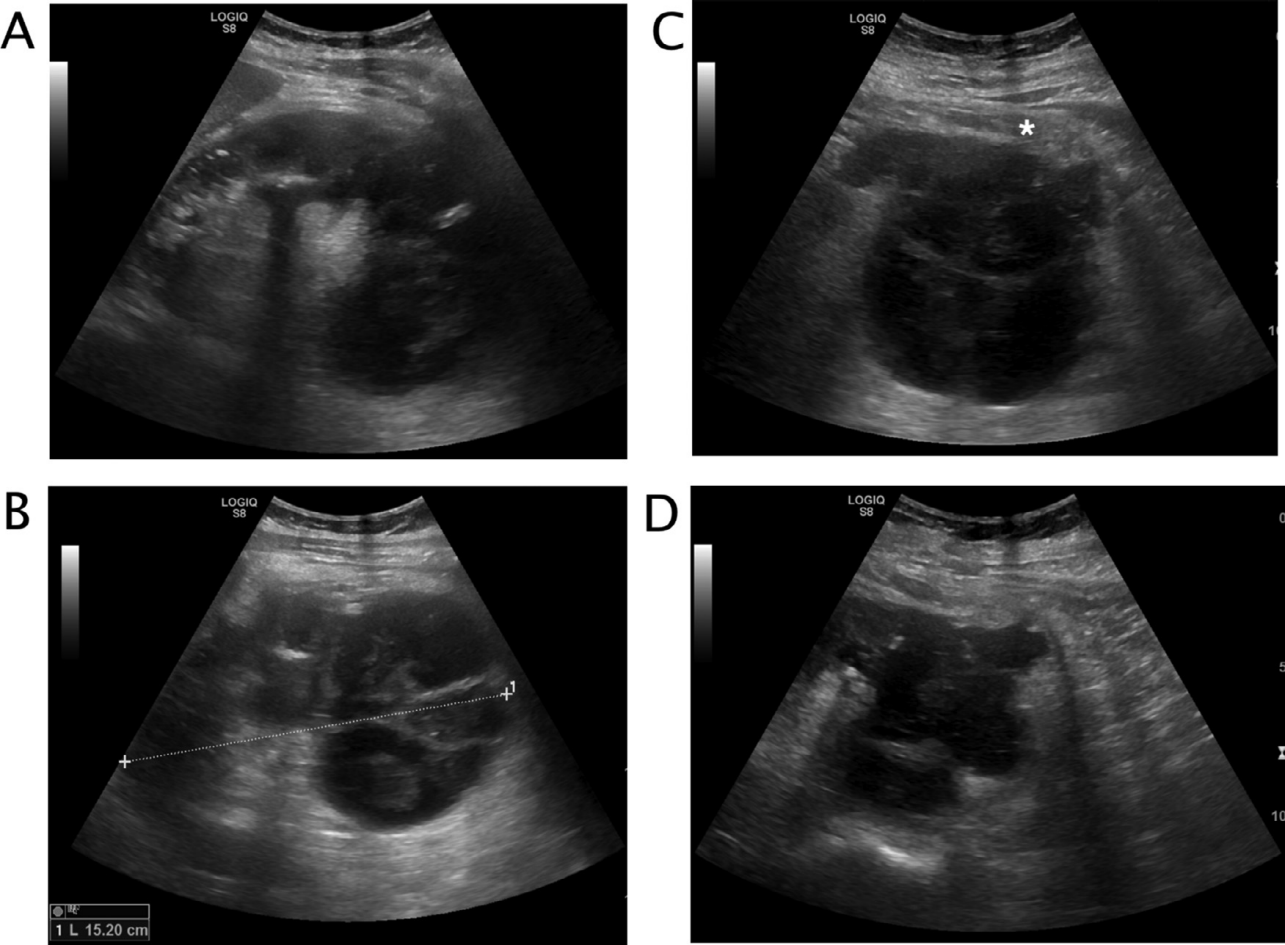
Left kidney ultrasound. The kidney is enlarged (A, B) with fluid filled dilated calico-pelvic system, within inhomogeneous hypoechogenic material is appreciable (A, C). Hyperechogenic spot are visible, referable to calculi (A, B). The outer border of the lower pole is irregular, and dilated calyceal system appear corticalized and protruding into the perirenal fat close to the perirenal fascia that appears thickened (*) (C, D). Perirenal fat is inhomogeneous and slightly hypoechogenic (D).
